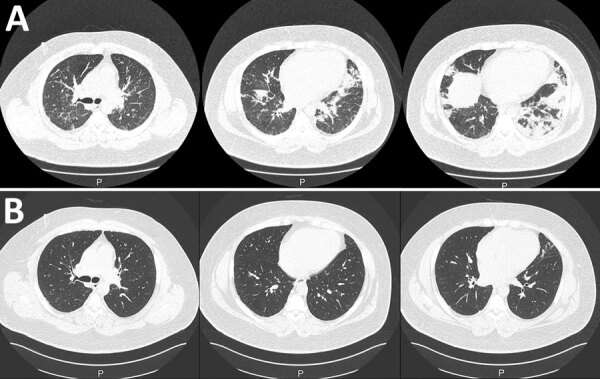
Chest computed tomography (CT) images of a male patient in Japan who was hospitalized with multisystem inflammatory syndrome. A) CT performed at hospital admission revealed infiltration in bilateral lower lobes. B) Chest CT performed 1 month after discharge revealed that most of these lesions had resolved.
